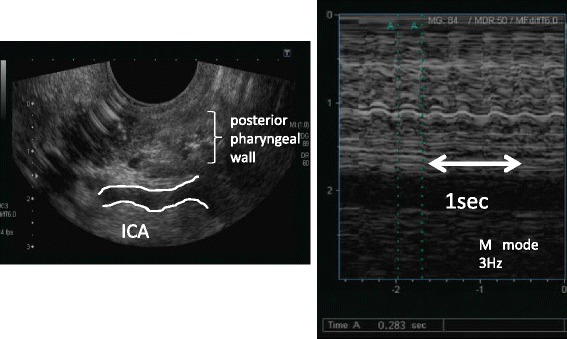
Measurement of frequency of the oral myoclonus with transoral carotid ultrasonography.
